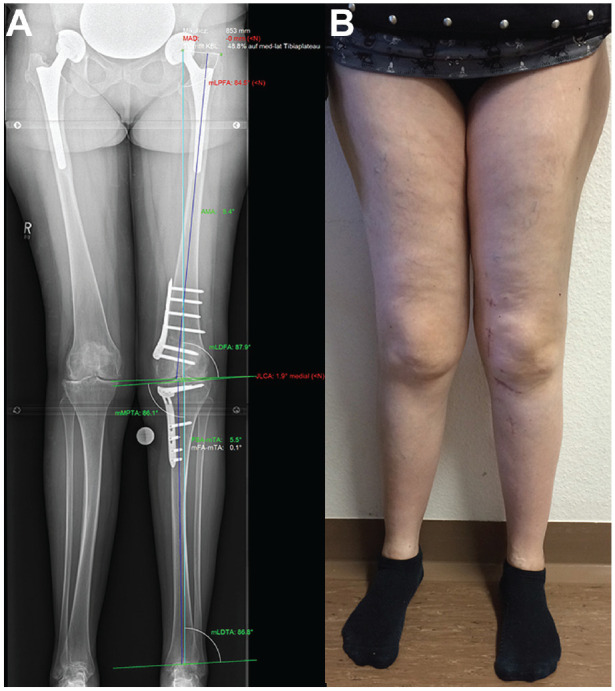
(A) Postoperative radiograph (mechanical tibiofemoral angle [mTFA], 0.1°; mechanical lateral distal femoral angle [mLDFA], 87.9°; mechanical medial proximal tibial angle [mMPTA], 86.1°) and (B) clinical photograph obtained in the same patient shown in Figure 1. JLCA: joint line convergence angle.
